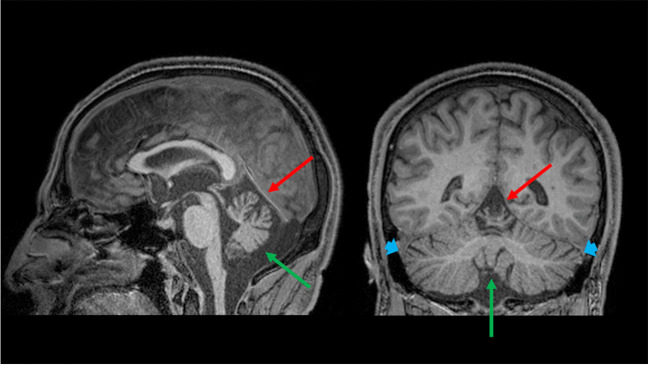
A pattern of selective cerebellar atrophy. Sagittal (*left*) and coronal (*right*) multiplanar reconstruction of a T1-weighted volumetric sequence of a 43-year-old male Autosomal Recessive Spastic Ataxia of Charlevoix-Saguenay patient showing a selective atrophy of the superior vermis (*red arrows*), with the remaining cerebellar structures (i.e., inferior vermis—*green arrows*—and cerebellar hemispheres—*blue arrows*) that appear unremarkable
